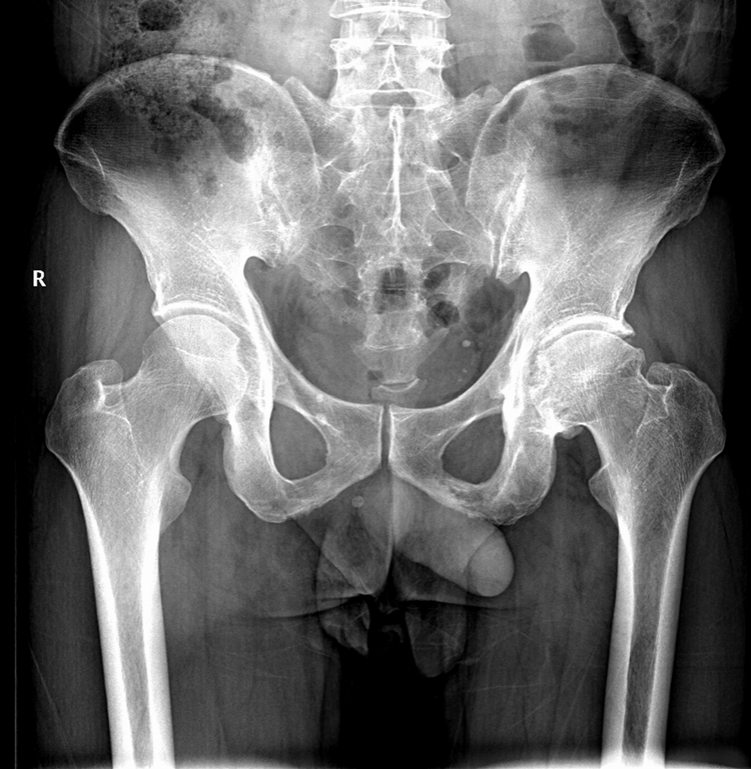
Plain radiograph of the pelvis shows deformation of the left femoral head, narrowing of hip space, and osteophyte hyperplasia.
